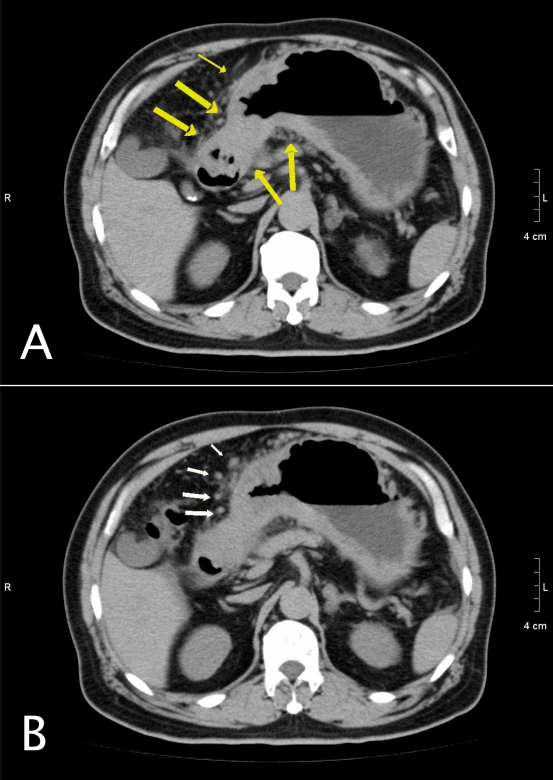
Complementary plain CT of the patient confirms thickening of the stomach wall. Adjacent peritoneal stranding and nodularity (arrows) is seen, which is suggestive of disease invasion.
